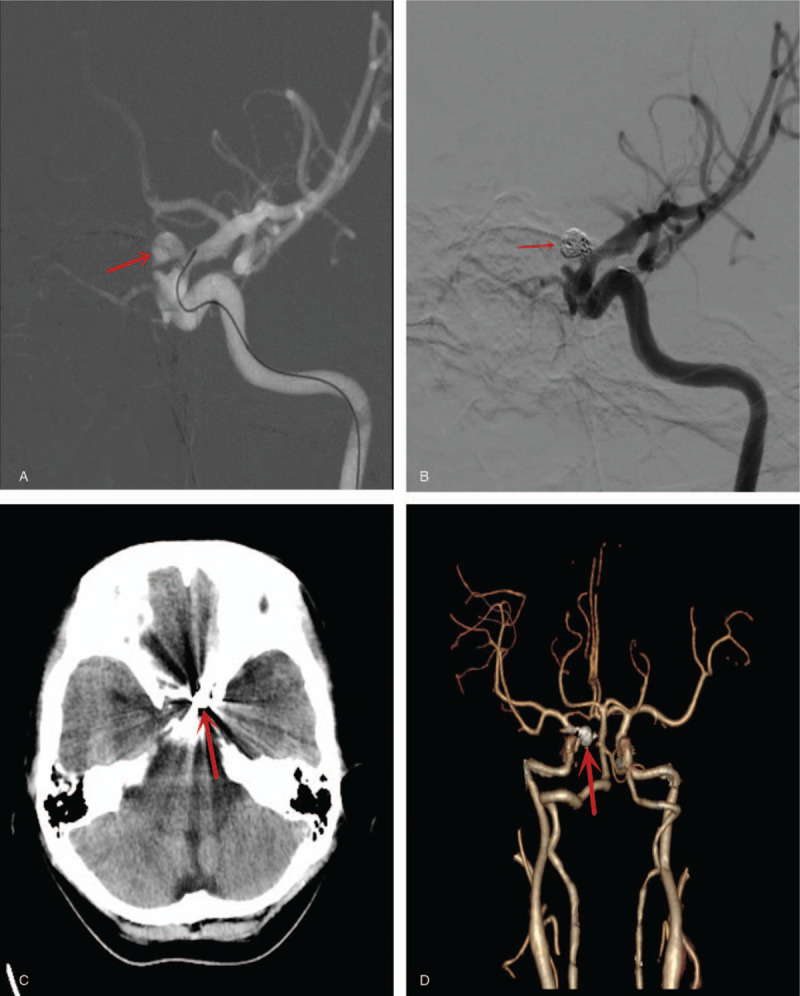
(A) Intraoperative digital subtracting angiography (DSA) image showing a dissected aneurysm in the ophthalmic segment of the left internal carotid artery (ICA) with an irregular neck as well as distal stenosis in the parent artery. (B) Intraoperative DSA showing occlusion of aneurysm with coils. (C) Postoperative computed tomography (CT) showing the opacity of the coils in-situ. (D) Postoperative CT angiography showing total occlusion of the aneurysm will coils.
